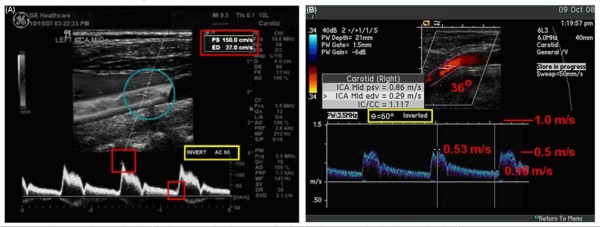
Normal CCA and ICA Images. A. This image was supplied to the UWURC on multiformat transparent film and scanned into the electronic image. Numbers in the red and yellow boxes have been enhanced for easier reading. B. The Doppler cursor is not aligned with the artery axis; the Doppler angle is 36°. A new velocity scale has been added in red along the right edge of the spectral waveform showing the results of the Doppler equation for a 36°angle.
